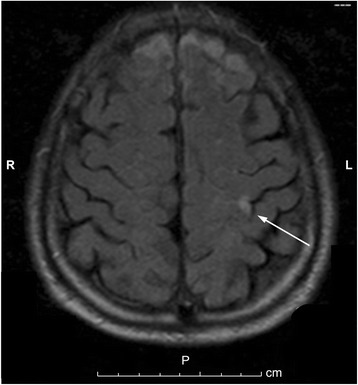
T2-weighted brain magnetic resonance image, showing an area of hyperintensity in the left precentral gyrus (*arrow*)
